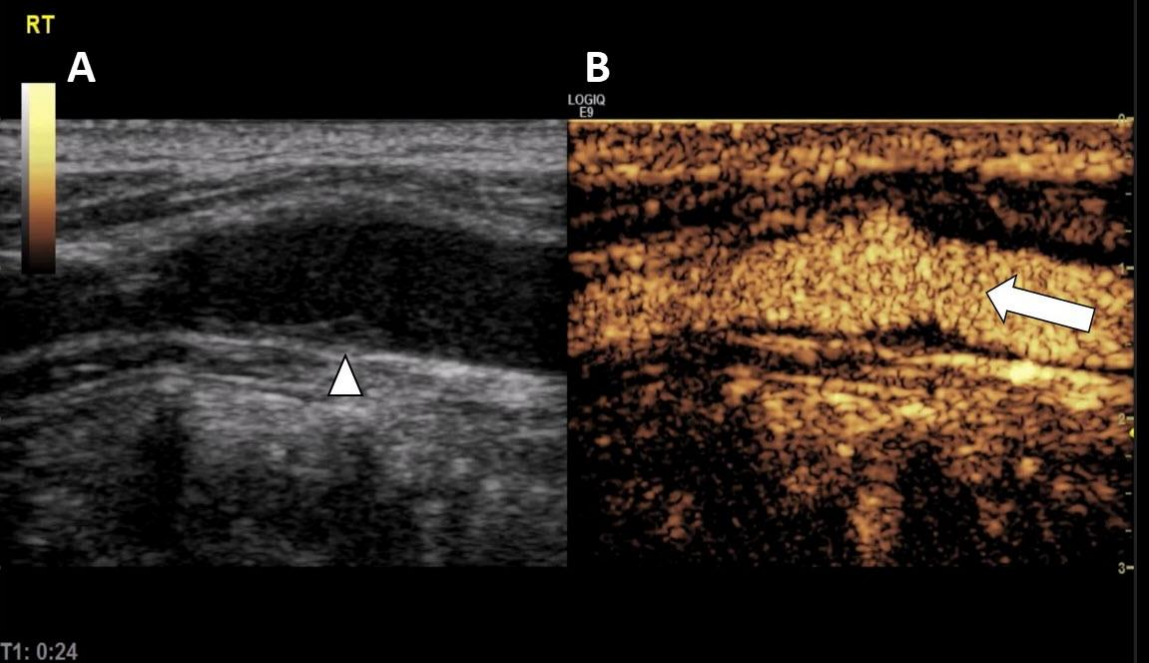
Contrast-enhanced Ultrasound (CEUS) Side by side: ultrasound grayscale image (A) and contrast-enhanced ultrasound image (B) of the carotid artery demonstrating the differentiation of the arterial wall atherosclerotic changes (arrow head) and blood flow in the vessel lumen (arrow).
